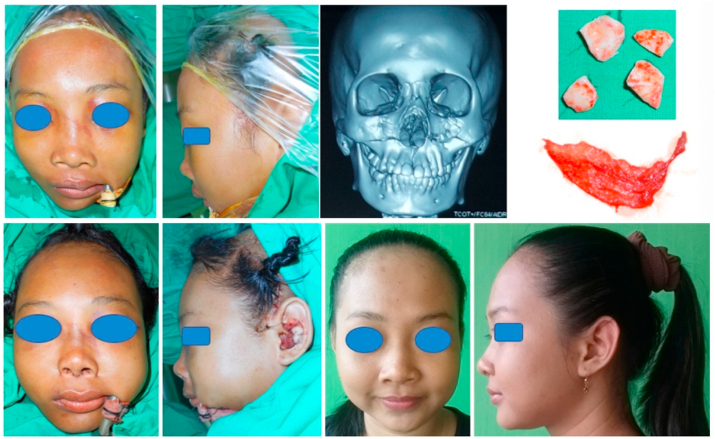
Above (Left to Right) Pre-op anterior view of the patient; Pre-op lateral view of the patient; Pre-op three-dimension CT scan of the patient; The conchal cartilage and superficial temporal fascia of the patient. Below (Left to right) Post-op anterior view of the patient; Post-op lateral view of the patient; Four years post-op anterior view of the patient; Four years post-op lateral view of the patient.
